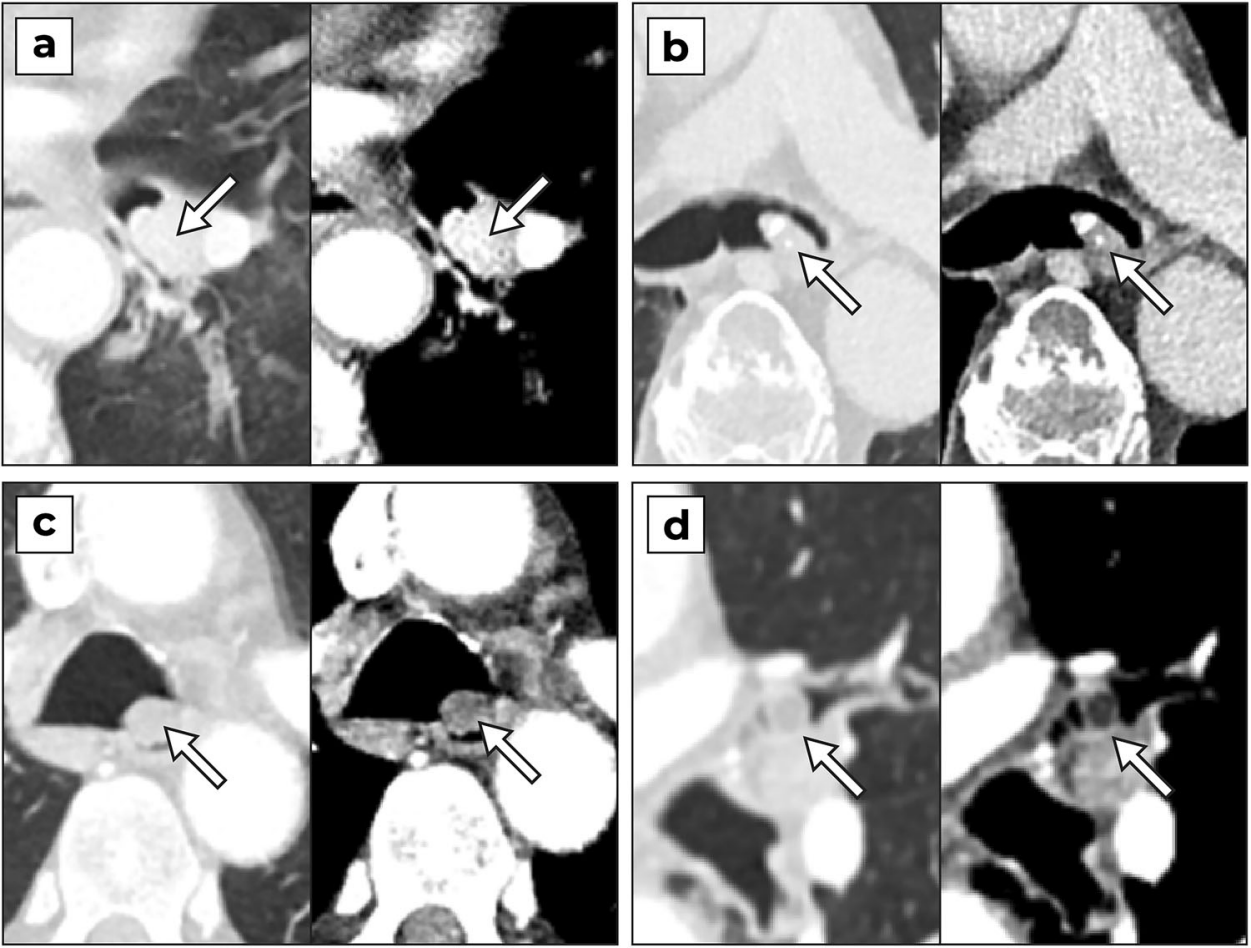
Examples of airway nodules in contrast-enhanced CT scans. For each panel, different window settings were applied for the lung (width: 1500 HU; level: −500 HU) and soft tissues (width: 350 HU; level: 0 HU). a A 60-year-old female patient with a carcinoid in the left lower bronchus (median attenuation value of 142 HU). b An 86-year-old male patient with a hamartoma with calcification in the left main bronchus (median attenuation value of 26 HU). c A 63-year-old male patient with nodular mucus plug in the distal trachea (median attenuation value of −18 HU). d A 57-year-old male patient with a lipoma in the left upper lobar bronchus (median attenuation value of −70 HU). HU, Hounsfield Units
